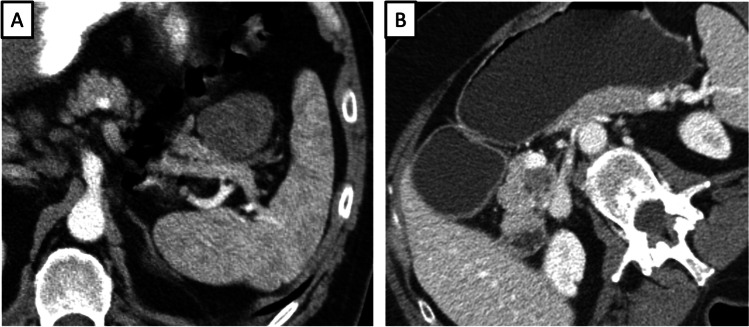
Comparison of lymphoepithelial cyst (LEC) and serous cystic neoplasm (SCN). (a) A 47-year-old male patient with LEC in the pancreatic tail, lesion size 4.4 cm. Axial CT image in the arterial phase (a) demonstrates the sponge-like appearance of LEC, mimicking SCN. (b) A 58-year-old male patient with small SCN in the pancreatic head. Axial CT image in the venous phase (b) shows a sponge-like cystic lesion in the dorsal part of the pancreatic head without signs of the infiltration of mesenteric vessels
